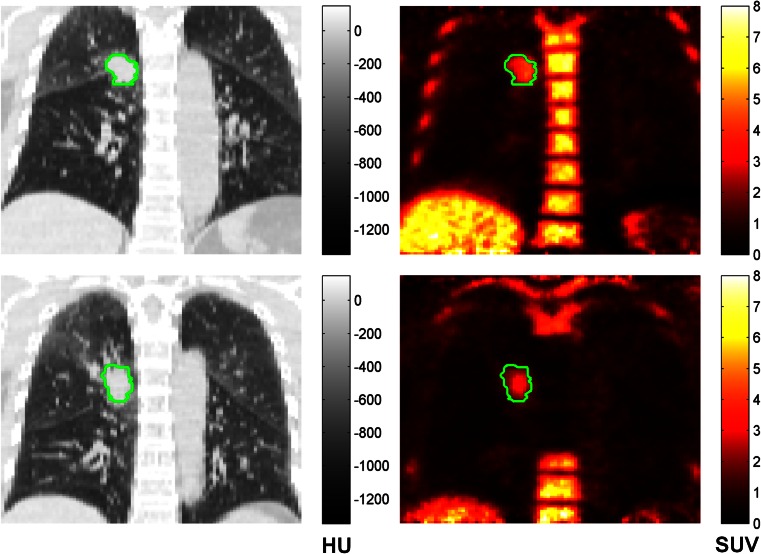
Representative CT (*left*) and PET (*right*, SUV 45–60 min) images of patient 11 acquired at baseline (*top*) and after delivery of 27.5 Gy (*bottom*). Primary tumour is manually delineated within *green outline*. Note sharply demarcated dramatic reduction in bone marrow uptake and modest reduction of tumour uptake after RT, as previously reported [21]
